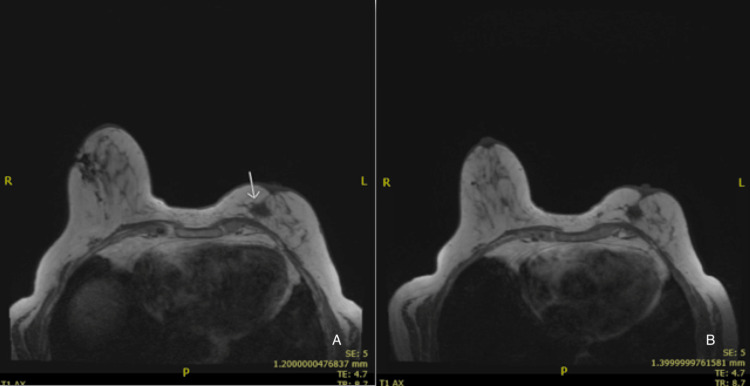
MRI findings. (A) Pre-chemotherapy. (B) Post-chemotherapy.
